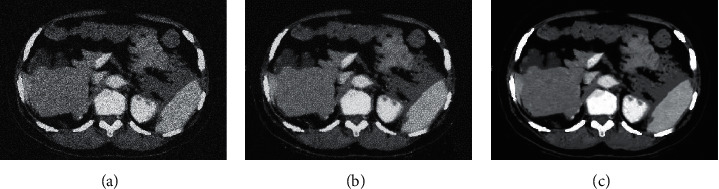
Comparison of CT images. (a) The image before CT enhancement. (b) The CT image after PSNR denoising. (c) The CT image after mean filtering algorithm denoising.
